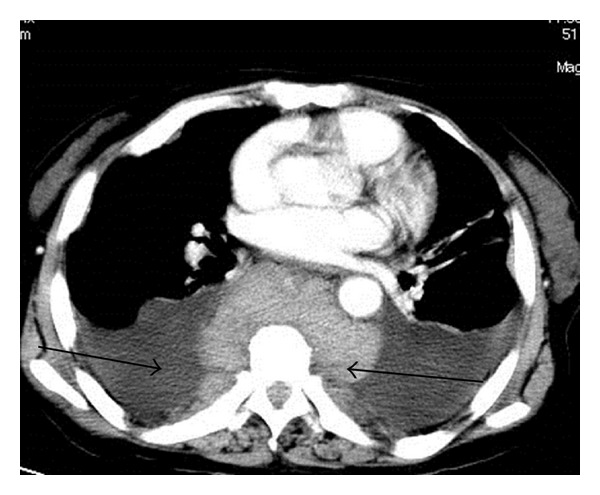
CT result suggests multiple round masses in the paraspinal and thoracic regions and bilateral pleural effusions. On November 13, 2009, CT scan was performed, and the left arrow indicated pleural effusion, and the right arrow suggested rounded soft mass surrounding vertebrae.
